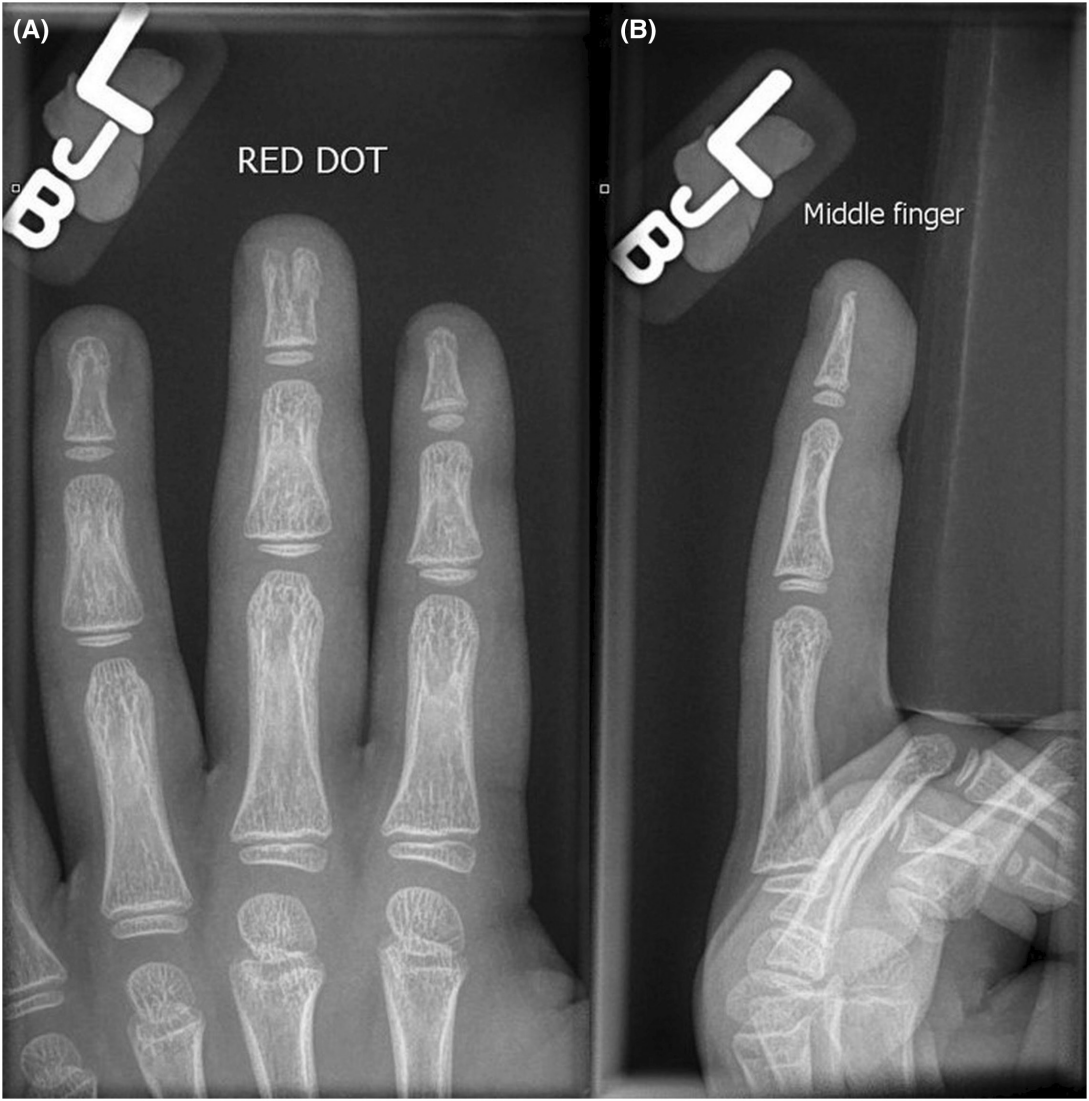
Anteroposterior (A) and lateral (B) view radiographs of the patient's left middle finger.
